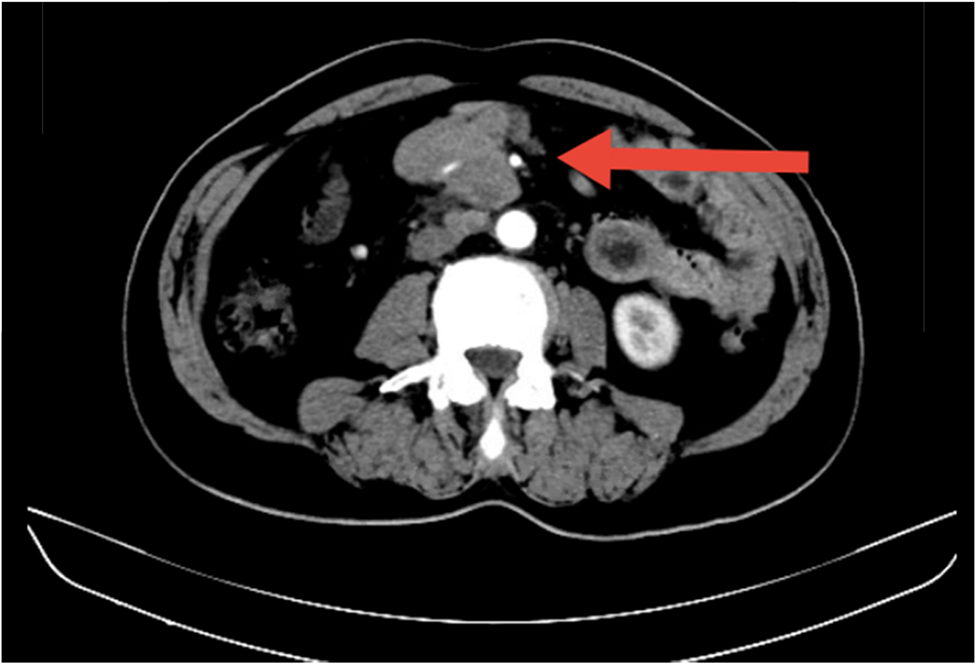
CT image revealed that the intestinal wall of some small intestine in the lower abdomen was significantly thickened and multiple lymph nodes were found in the retroperitoneal space (arrowhead).
